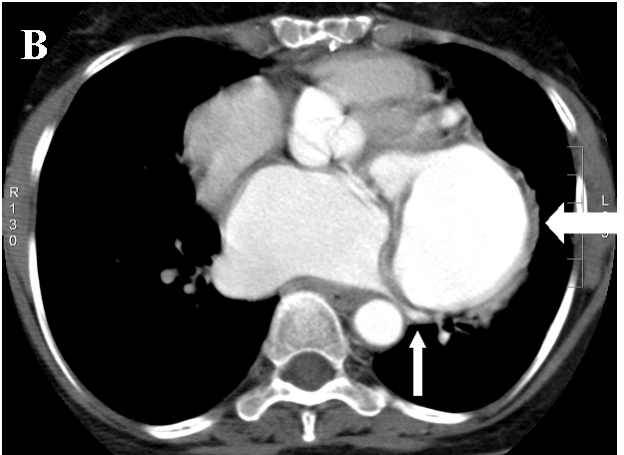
Axial source image of CT angiography showing the aneurysm (thick white arrow) compressing the left lower pulmonary vein (thin white arrow).
